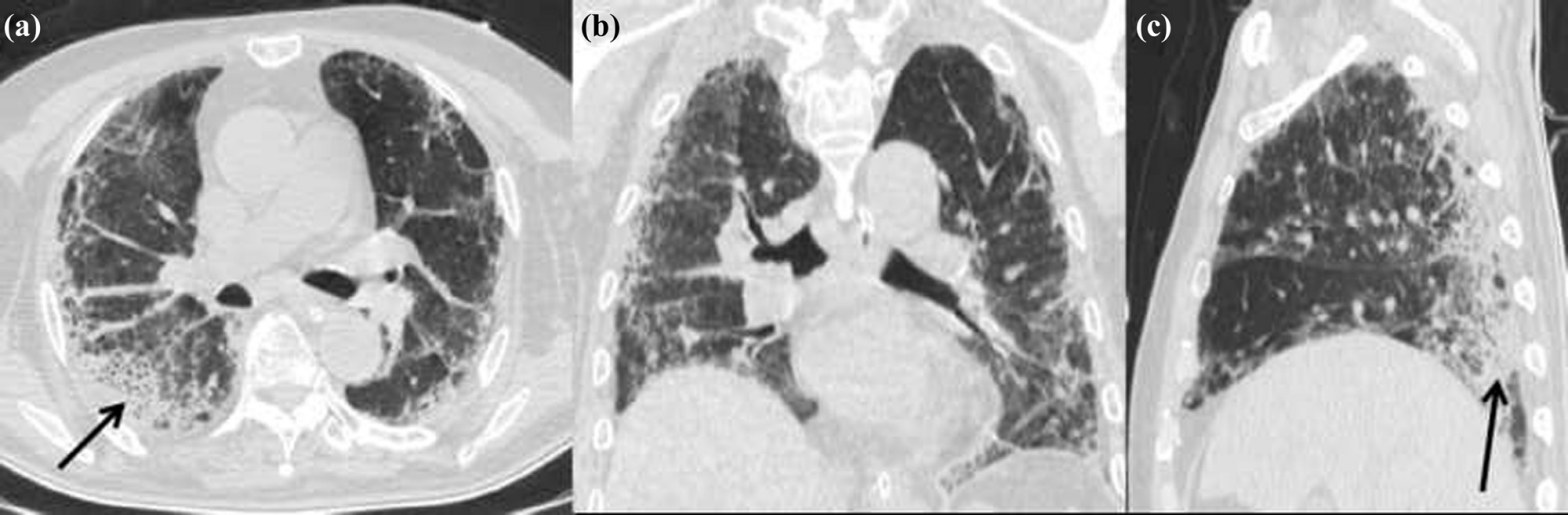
CT scans obtained 16 days after admission (a: axial non-contrast CT image; b: coronal thin-section noncontrast CT image; and c: sagittal thin-section noncontrast CT image) showing the range of lesions reduced compared with the previous images; partial patchy consolidation (black arrows).
